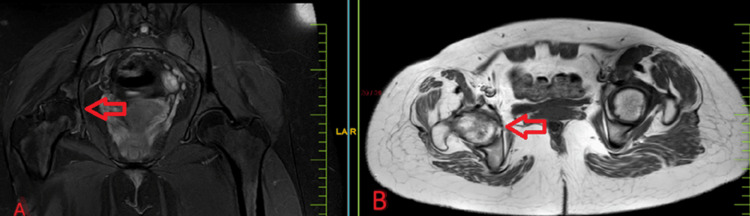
MRI pelvis with the hip A) coronal section and B) axial section showing that the right femoral head is seen slightly impacted in the left acetabulum causing surface irregularity of both the femoral head and acetabular cavity with loss of sphericity. Reactive edema is also seen in the acetabulum, and a crescent sign is noted in the right femoral head. These features suggest avascular necrosis of the right femoral head and Ficat and Arlet stage III classification. MRI: magnetic resonance imaging
